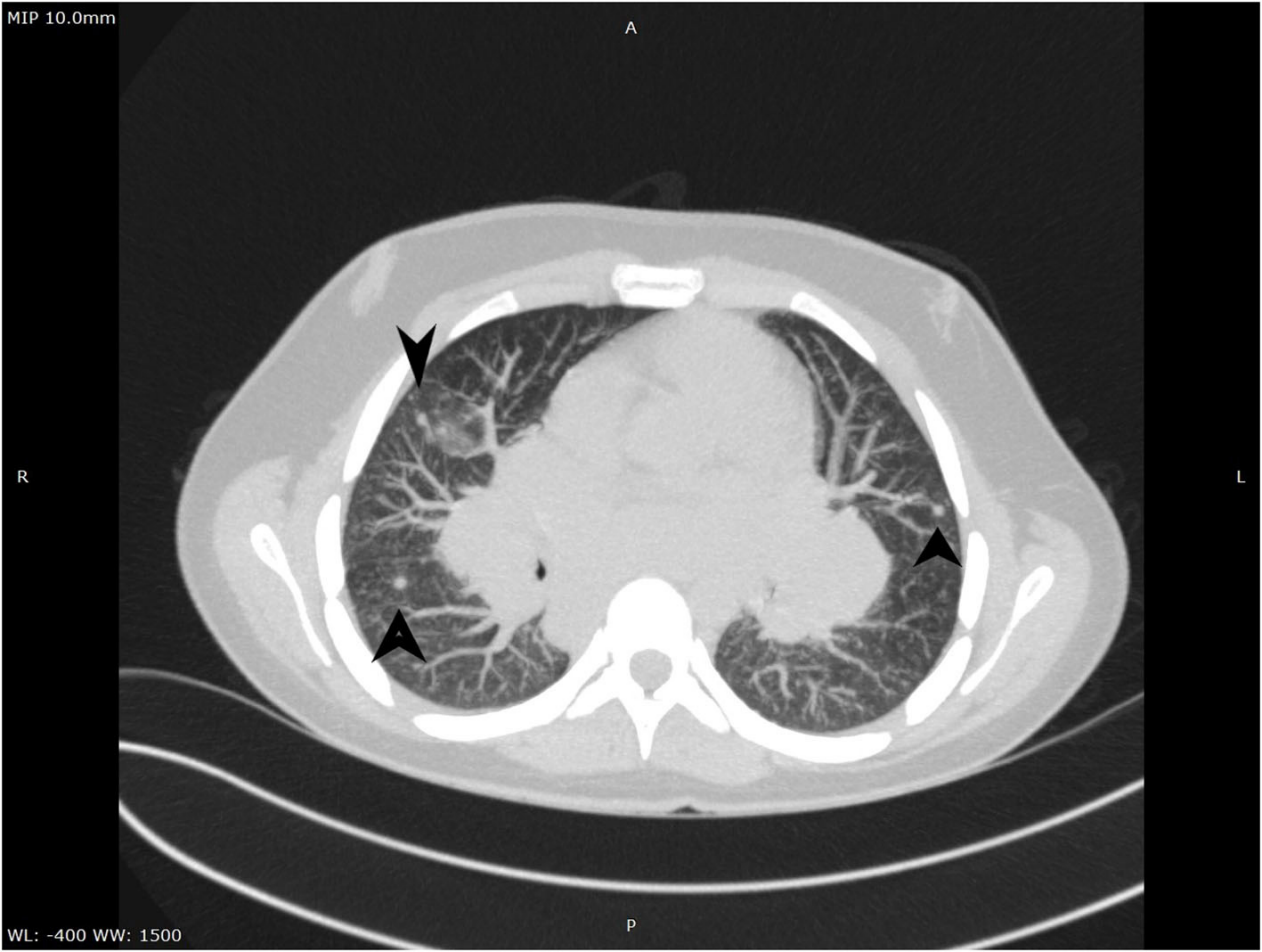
Ground-glass aspect on CT.
